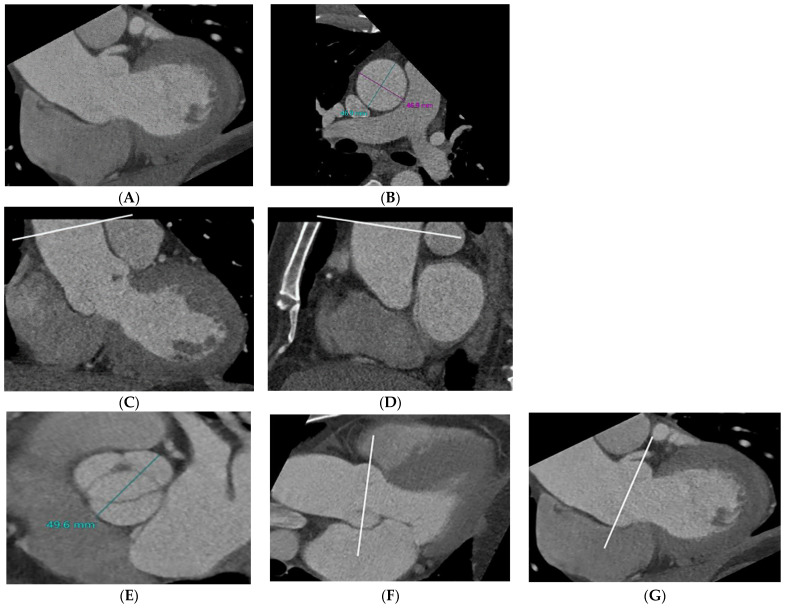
Example of the measurement of the aortic dimensions at different locations. Oblique sagittal reformatted CT image shows the sinus of Valsalva and tubular portion of the ascending aorta at mid-systole (A). The maximum dimension of the tubular portion of the ascending aorta (white line) was measured in a double-oblique transverse view (B) obtained perpendicular to the aortic lumen (C,D) because the axial measurement exceeded the true measurement perpendicular to the long axis of the ascending aorta. The maximal aortic root dimension (white line) was measured sinus-to-sinus in a bicuspid aortic valve with a raphe (E) in a double oblique transverse view obtained perpendicular to the aortic sinus of Valsalva (F,G).
